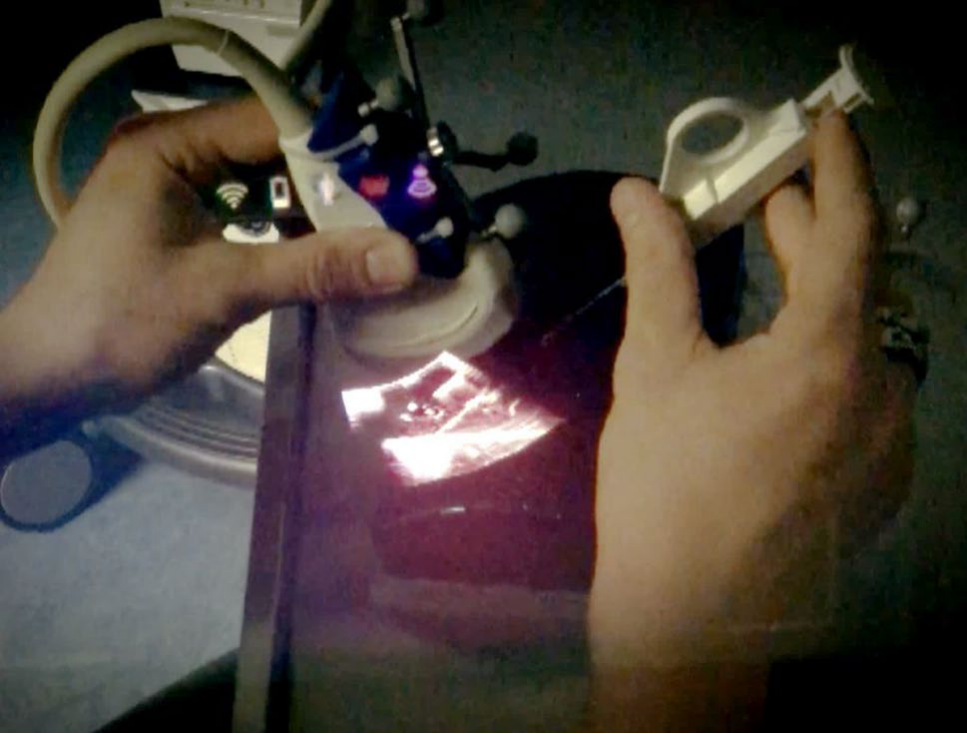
Ultrasound-guided needle placement with image overlay at the transducer (image was captured through the HMD resulting in limited resolution; the actual overlay accuracy was higher)
